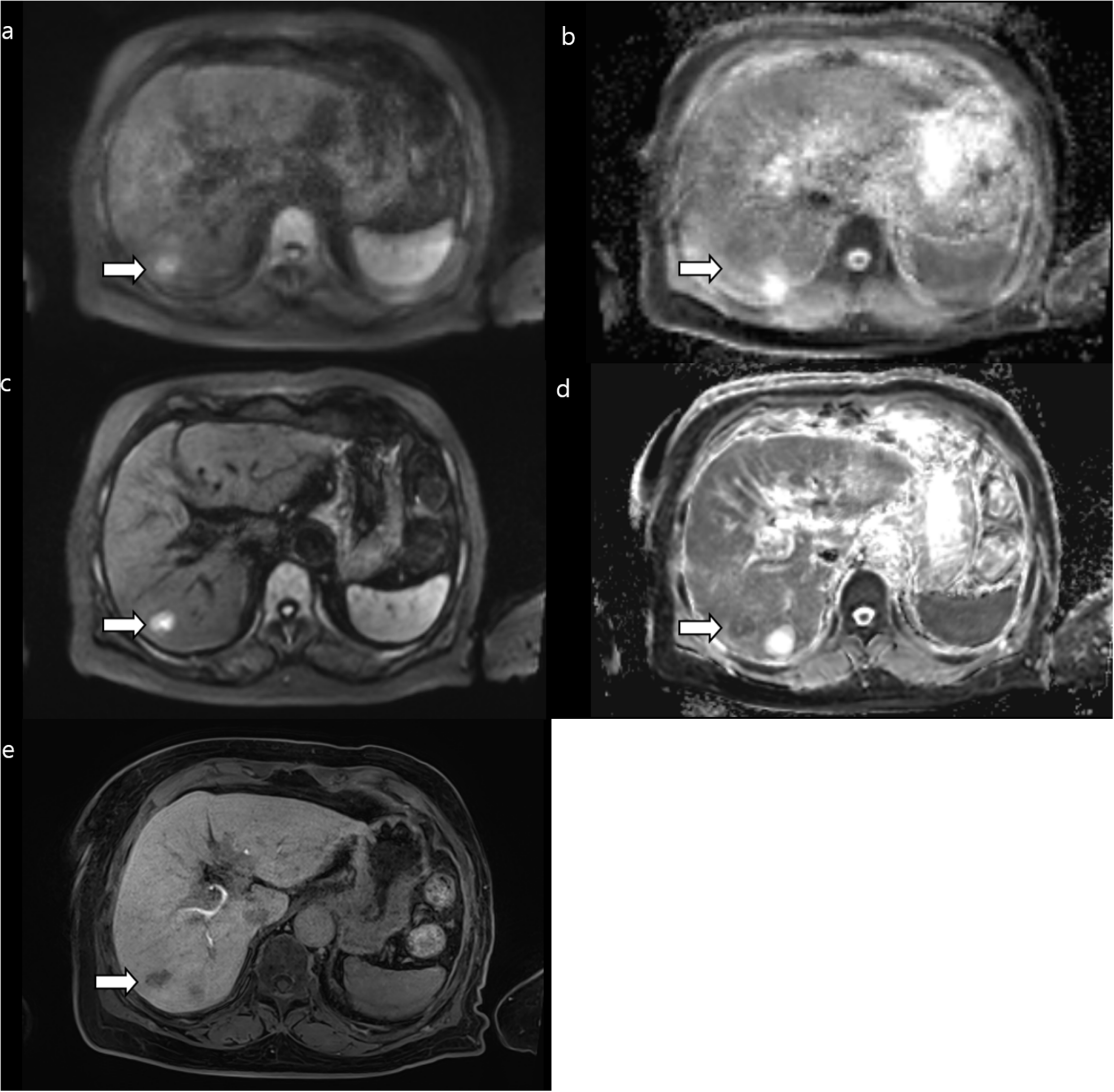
A 2-cm TACE indicates a hepatocellular carcinoma (arrows) in segment 7 in a 50-year-old female patient showing severe motion artifacts on free-breathing, conventional DWI (FB-C-DWI) (a) and the ADC map (b). There are no motion artifacts on free-breathing DWI using a DL reconstruction (FB-DL-DWI) (c) and on the ADC map (d). The defect in the hepatobiliary area is evident (e). ADC, apparent diffusion coefficient.
